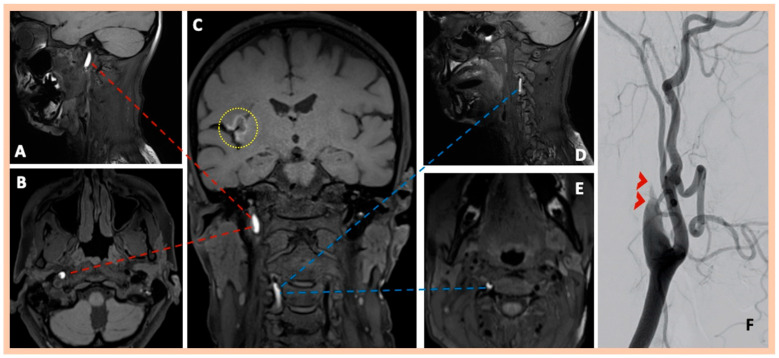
Neuroimaging findings—Patient #1. Three-dimensional fat-saturated black-blood T1-weighted high-resolution (3 Tesla) vessel wall imaging with multiplanar reconstruction, showing a characteristic (crescent-shaped), hyperintense lesion in the vessel wall of the right internal carotid artery, indicating intramural hematoma (Panels (A): Sagittal—(B): Axial—(C): Coronal; red dotted lines). Moreover, an asymptomatic spontaneous dissection with intramural hematoma along the distal intraforaminal (V2) segment of the right vertebral artery was detected using the same MR sequence (Panels (C): Coronal—(D): Sagittal—(E): Axial; blue dotted lines). The subacute right middle cerebral artery infarction is also depicted in the T1-weighted SPACE images. (Panel (C)—yellow circle with dotted lines). Digital subtraction angiography confirmed the right ICA dissection, revealing a “flame-shaped” occlusion (Panel (F)).
